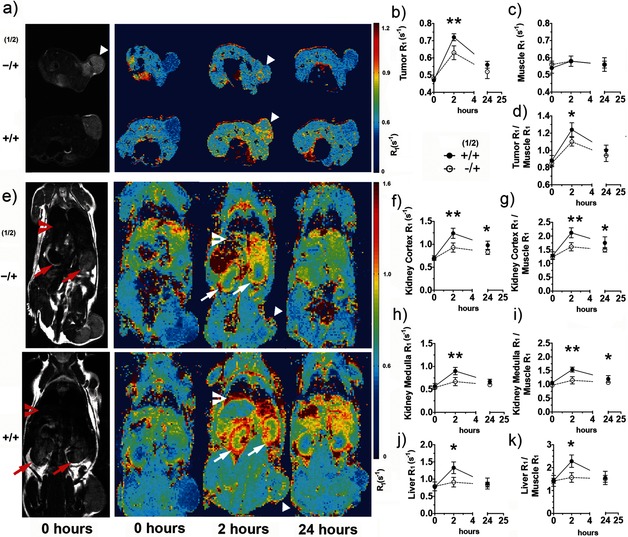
Imaging tissue glycosylation in vivo using MRI *T*
